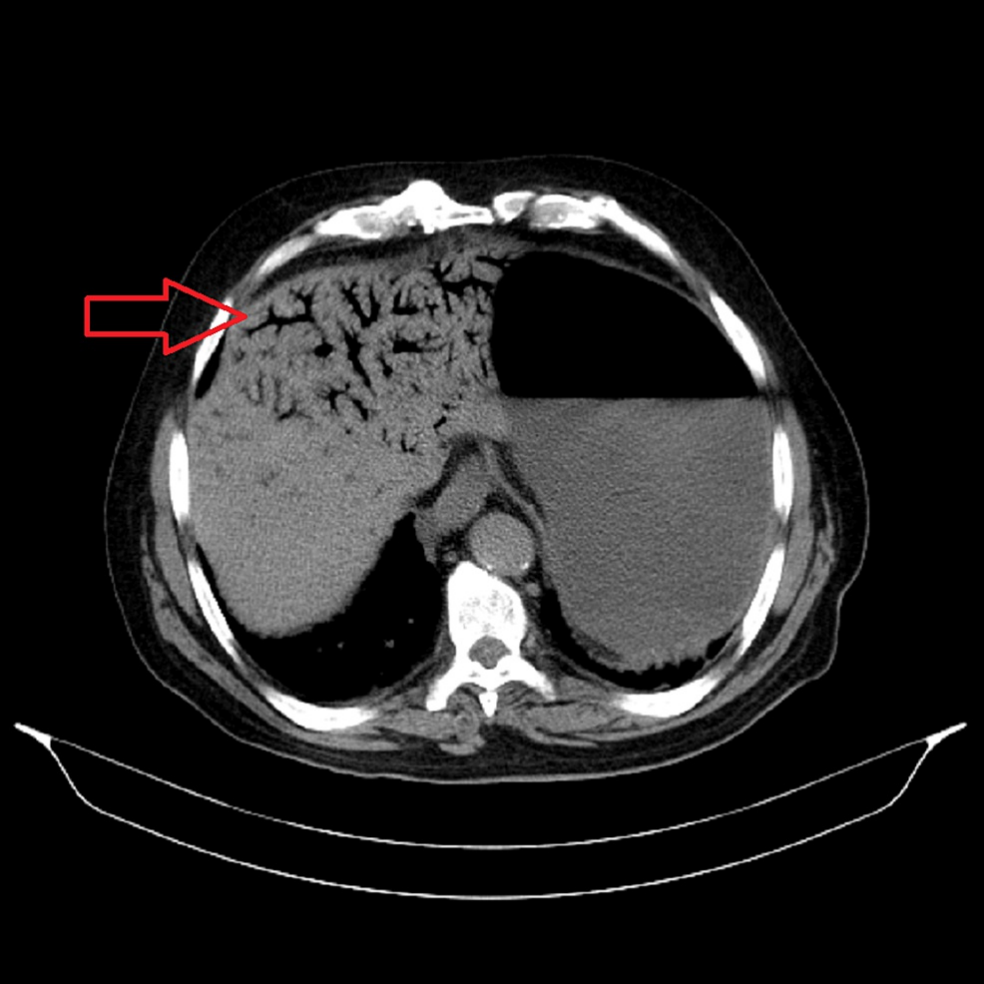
CT scan of the abdomen showing aeroportia (arrow)
